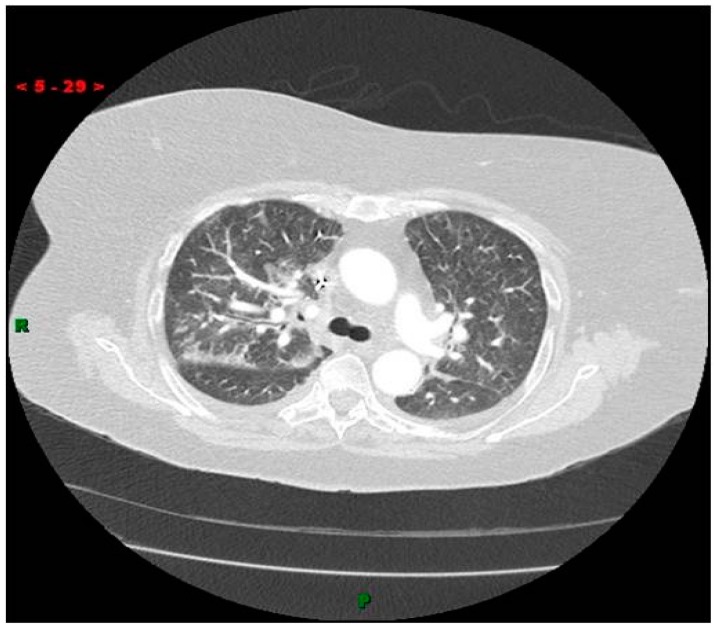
Computed Tomography (CT) scan of the thorax showing numerous pulmonary radiodensities with suspected cardiac radio-densities.
